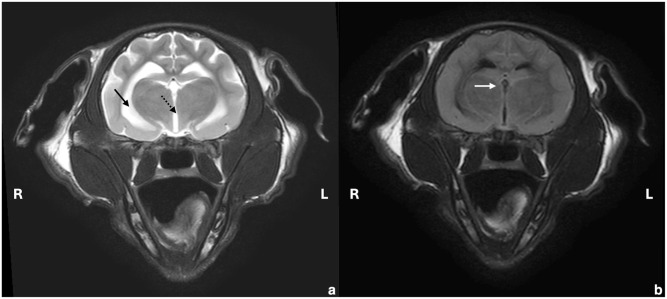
Transverse (a) T2-weighted and (b) T2-fluid attenuation inversion recovery (FLAIR) images of the brain at the level of the thalamus and third ventricle. The ventricular system is moderately widened (arrow: lateral ventricle, dashed arrow: third ventricle) with incomplete cerebrospinal fluid suppression in the FLAIR sequence (white arrow)
